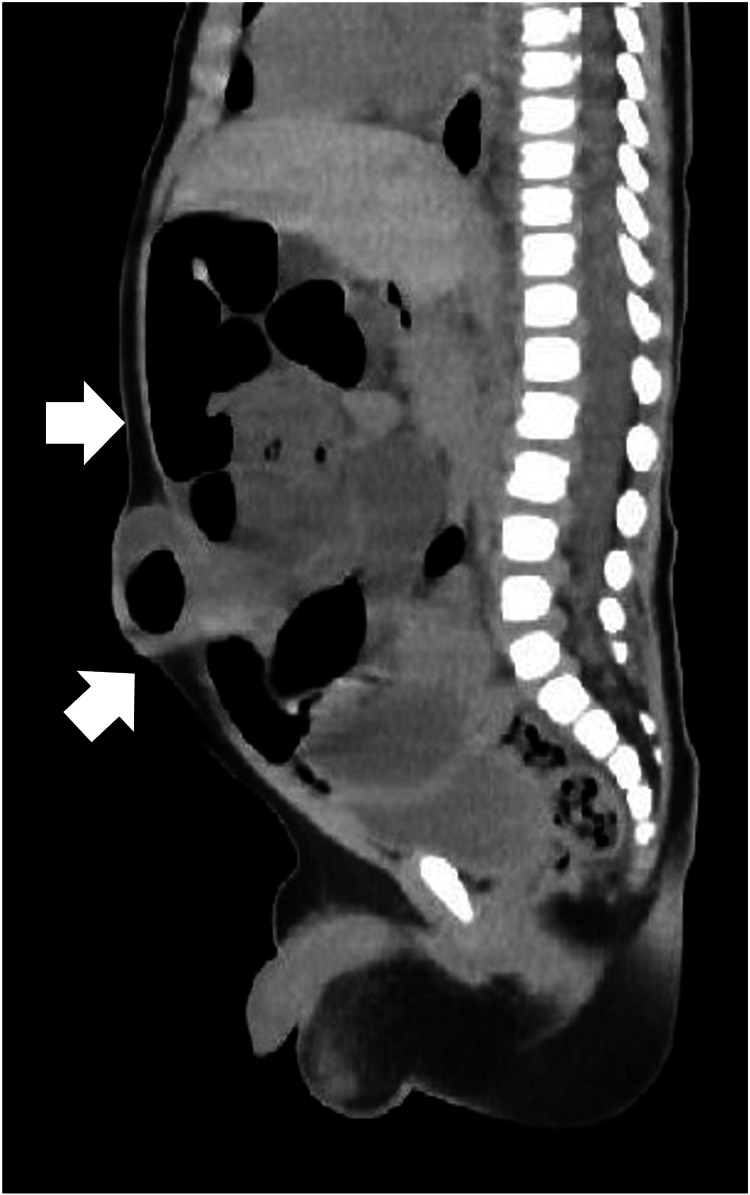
Abdominal computed tomography showing the prolapse of the UH with dilated intestines
